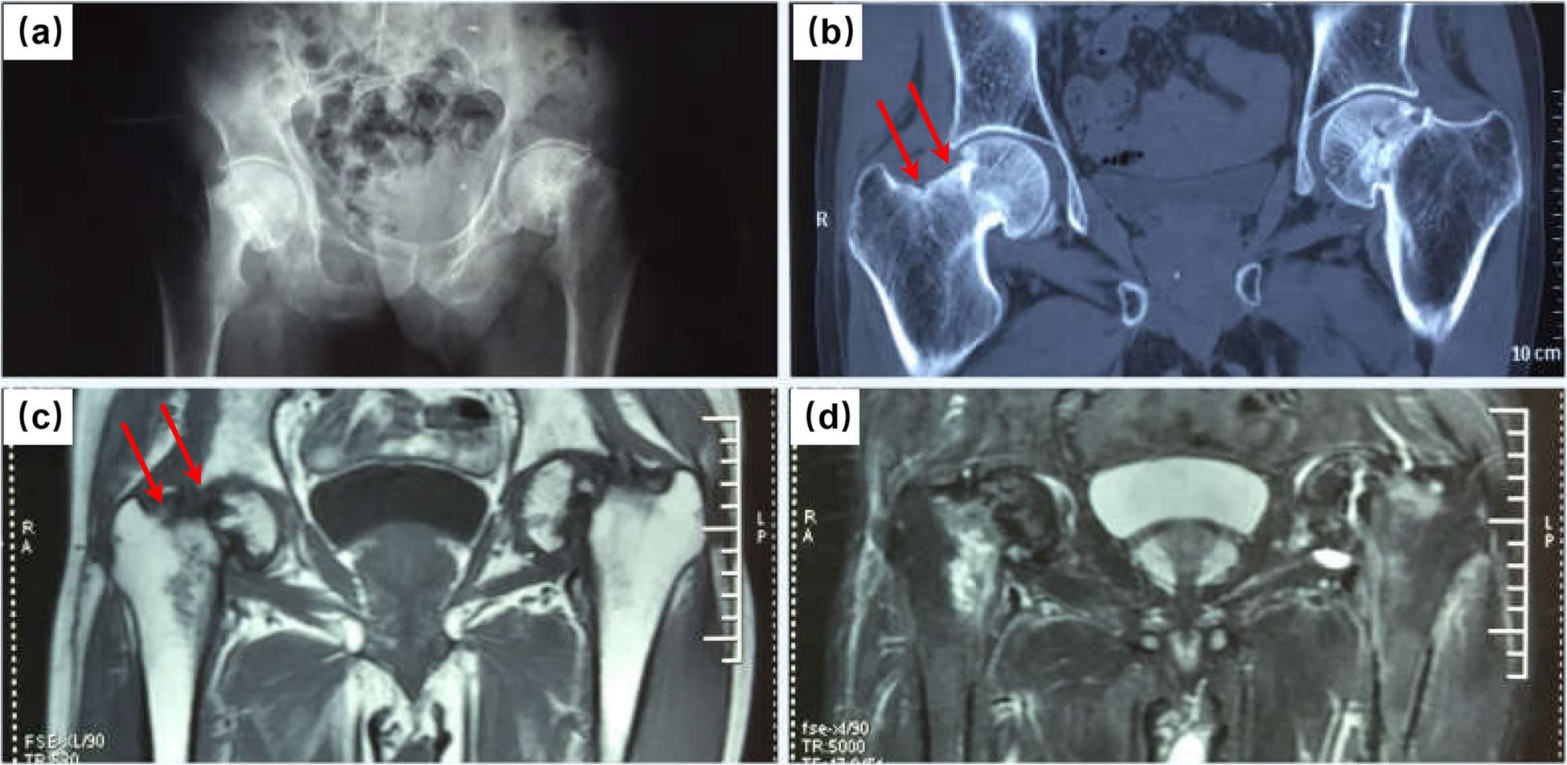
Imaging examinations of the patient in the hip region after admission into our hospital. a Plain radiograph of the pelvis indicating fracture of left femoral neck, with obvious displacement, nonunion and femoral neck shortening, and old fracture of the right femoral neck. b CT image of the coronal plane revealing bilateral femoral neck insufficiency fractures, with obvious displacement, nonunion and femoral neck shortening in the left femoral neck and double fracture line (arrows) in his right femoral neck. c Coronal T1-weighted image showing low signal intensity in the fracture region of bilateral femoral neck, with double fracture lines (arrows) on the right side. d Coronal T2-weighted image showing swelling on the right femoral neck and interruption of cortex on bilateral femoral neck
